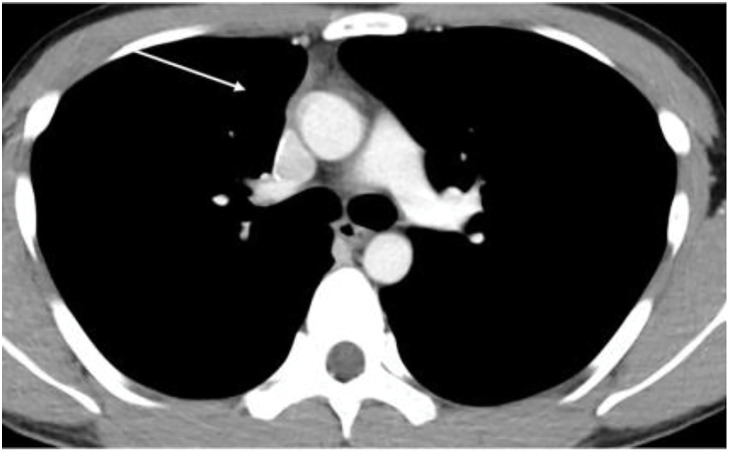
Chest computed tomography findings showing complete tumor disappearance.
